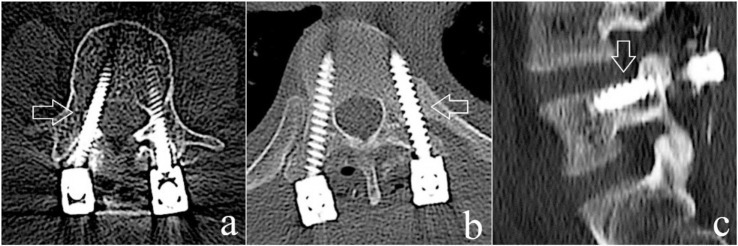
Postoperative CT image showing various directions of pedicle screw breach. a) Medial; b) Lateral; c) Superior.
